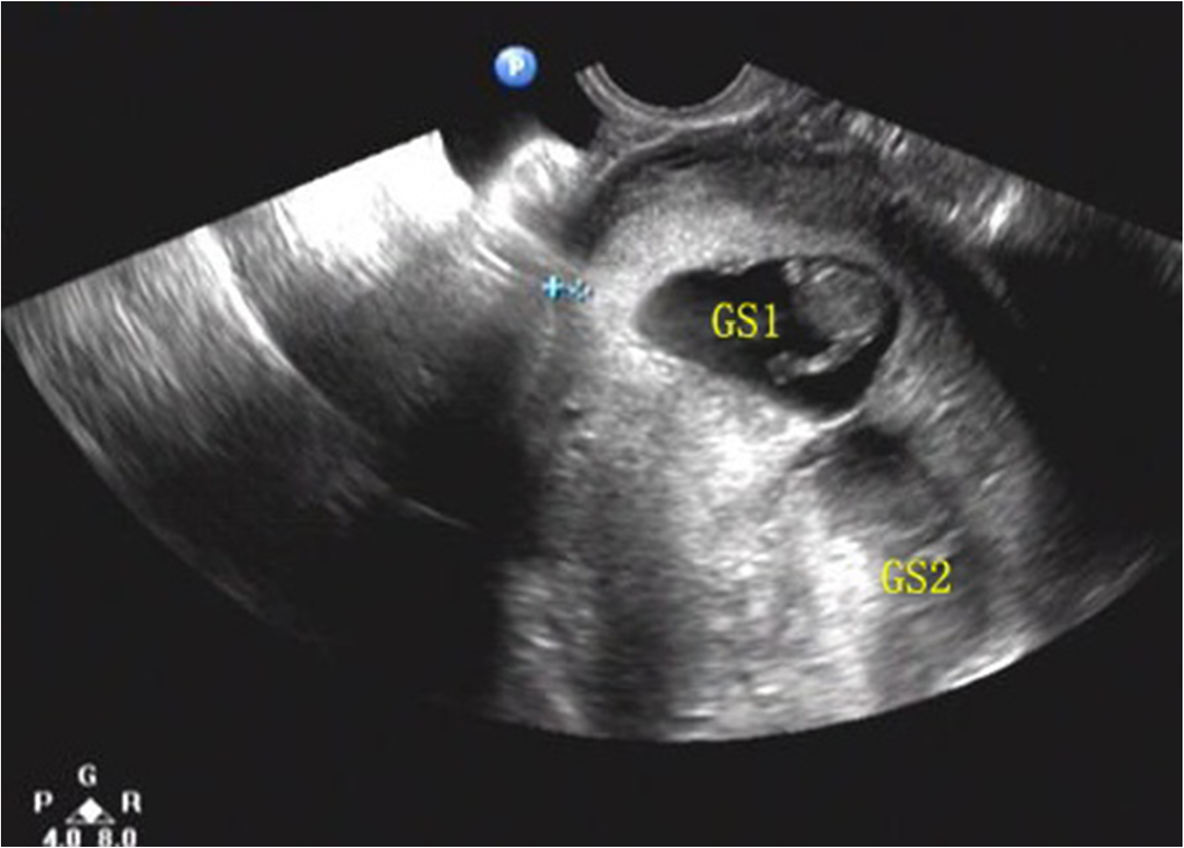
Diagnosis of cesarean heterotopic pregnancy via transvaginal ultrasonography at 11 + 1 weeks’ gestation. Myometrial layer of cesarean scar 4 mm
